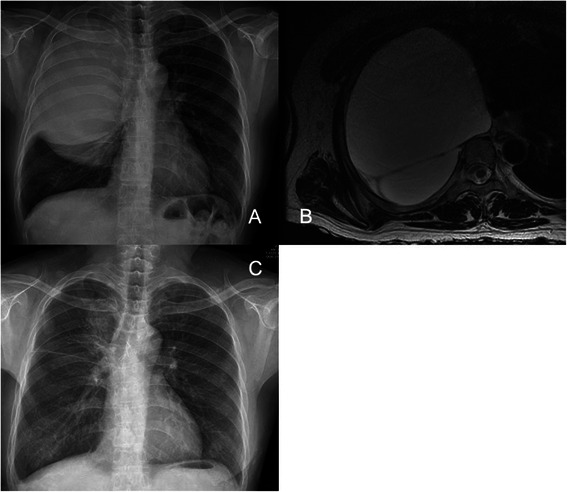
A plain chest X-ray (a) and magnetic resonance images show huge cystic masses on the right hemithorax continuing at the spinal canal (b). Post-operative plain chest X-ray (c) with a follow-up in 8 months after surgery: shows a markedly decreased meningocele of the posterior mediastinum and no sign of cerebrospinal fluid leakage
